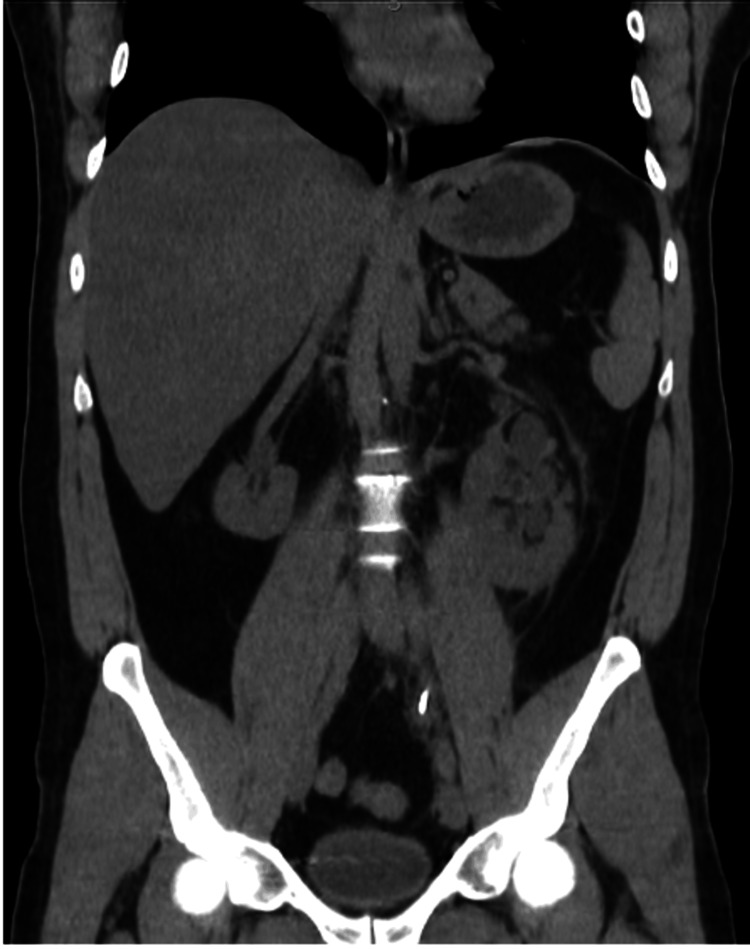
Postoperative nonenhanced CT Twenty-four hours after surgery, a non-enhanced CT scan showed a stone-free-rate status.
